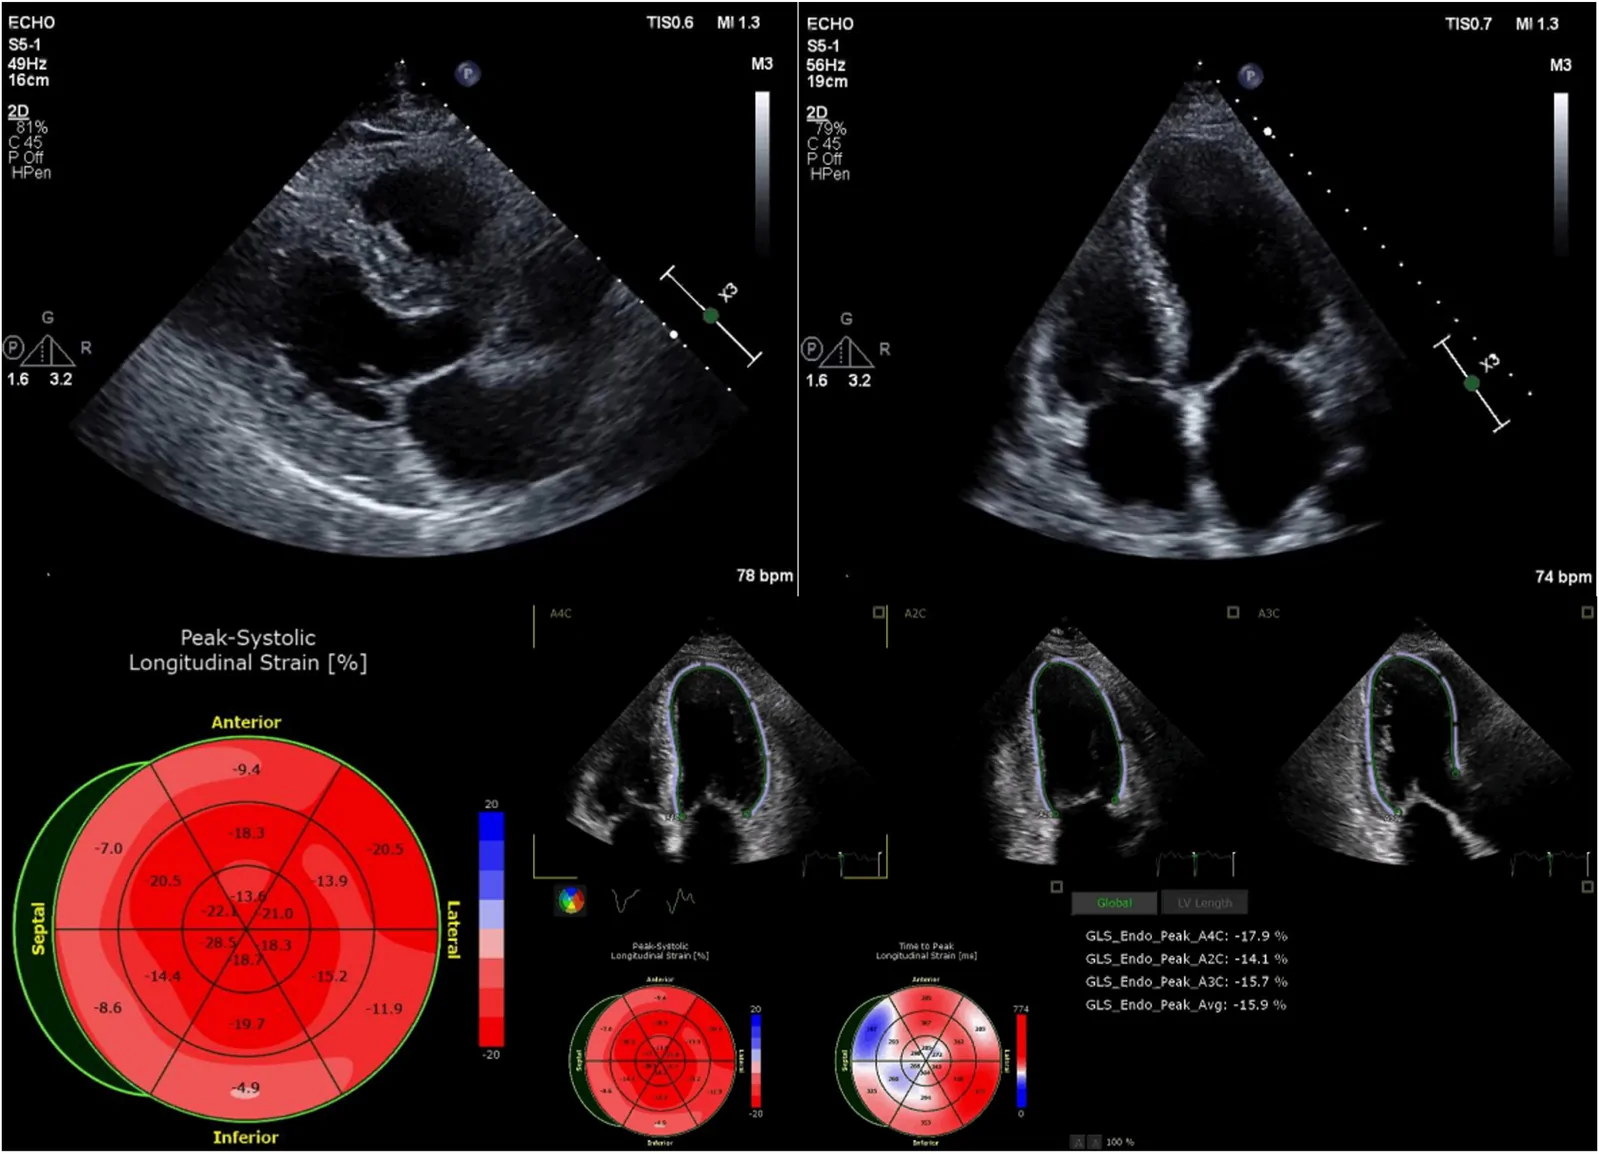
Transthoracic Echocardiogram Transthoracic echocardiogram demonstrating parasternal long axis (top left) and apical 4-chamber (top-right) views. Global longitudinal strain with reduced strain (−15.9%) and relative apical sparing (so-called cherry on top pattern) is consistent with features of cardiac amyloidosis (bottom panel).
